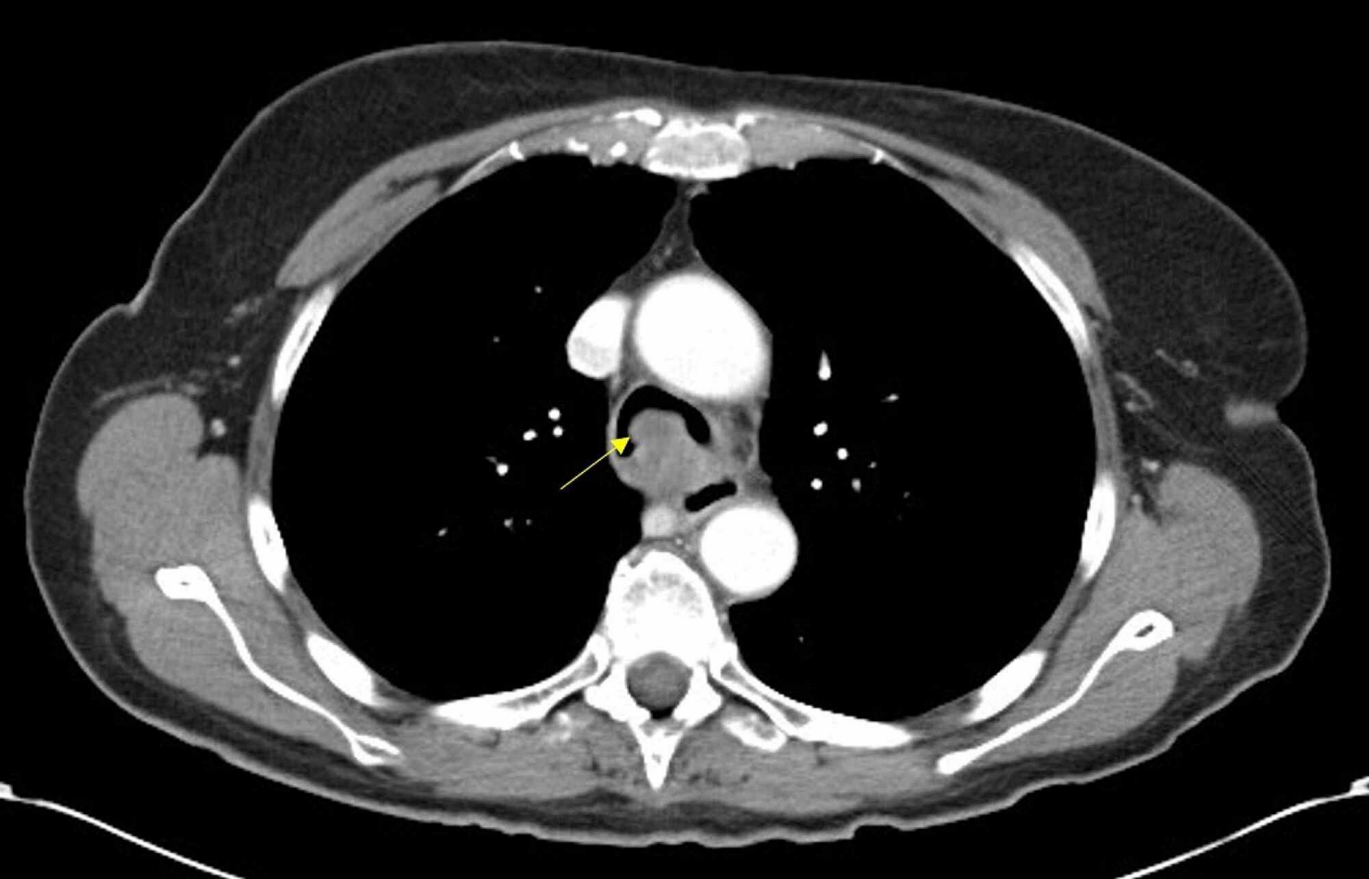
Axial cut of CT Chest showing luminal narrowing due to tracheal mass.
